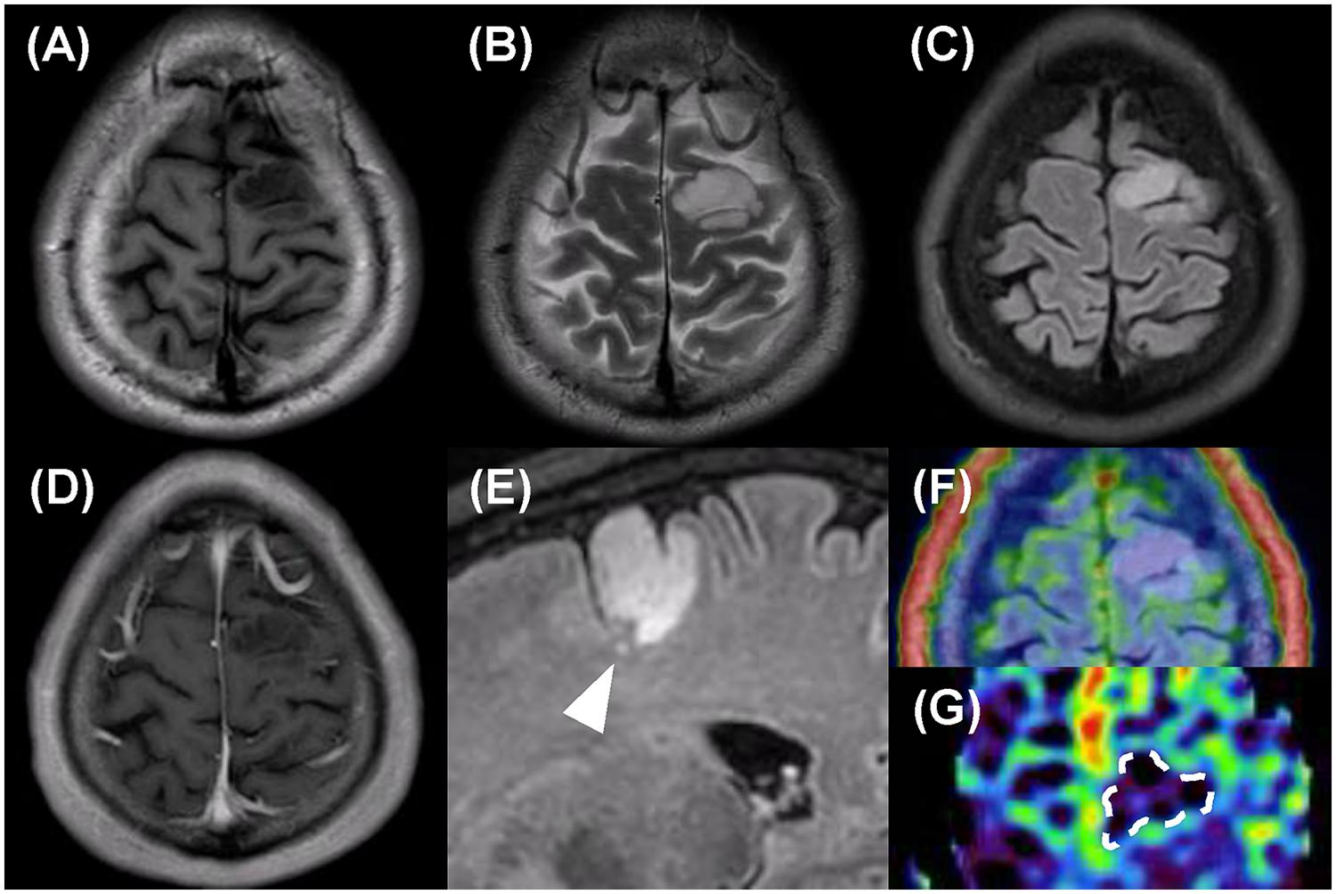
Findings on initial brain magnetic resonance images. (A) Axial T1-weighted image showing heterogeneous hypointensity in the precentral gyrus. (B) Axial T2-weighted image showing heterogeneous hyperintensity. (C) Axial FLAIR image with the same findings as those on the T2-weighted image. (D) Axial T1-weighted image with contrast showing no enhancement. (E) A sagittal thin-slice FLAIR image showing the hyperintense lesion and one hyperintense nodule (arrowhead) in the white matter without mass effect. (F)
